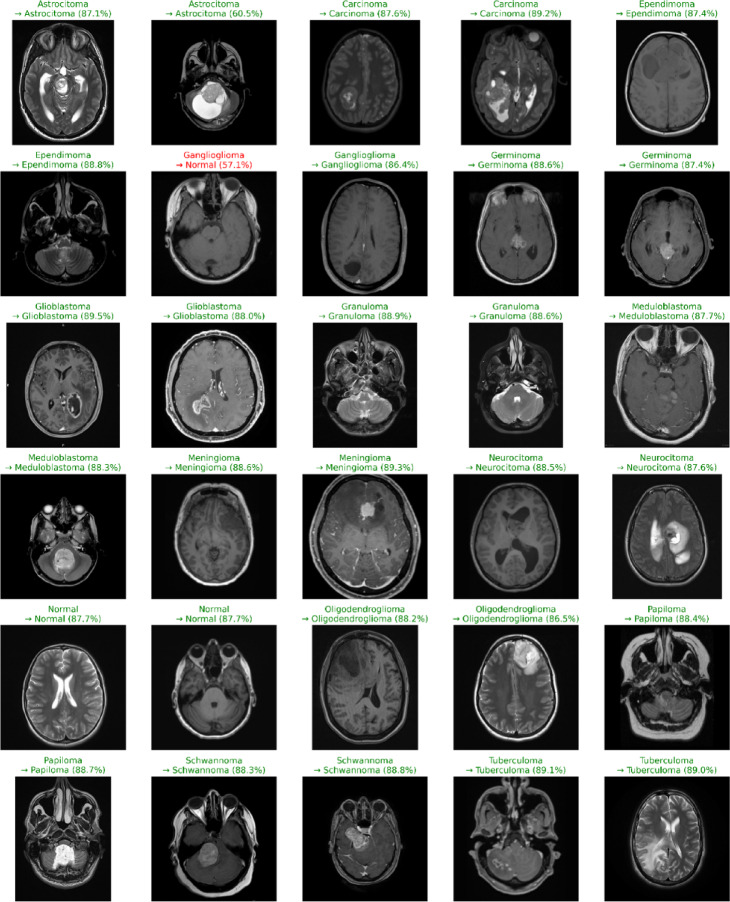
Classification results of brain MRI test images using the proposed algorithm.
